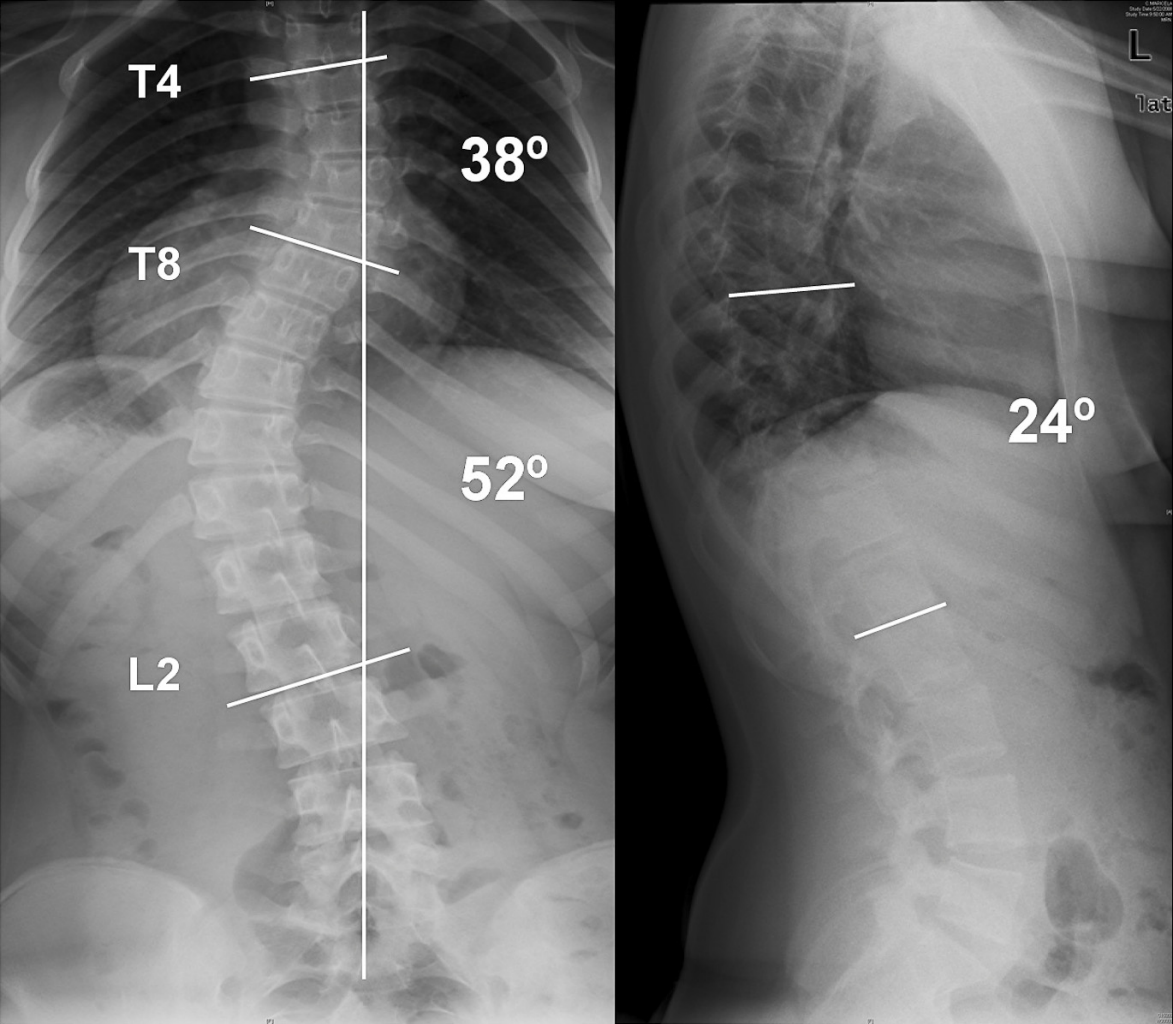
"Patient 1 - Anteroposterior and lateral preoperative radiographs." Patient 1 - Anteroposterior and lateral radiographs show a 52° main thoracic curve and a 38° upper thoracic curve, with 24° dorsolumbar kyphosis. MIS technique was utilized (Figure 10 & 11), and both of the curves were instrumented.
